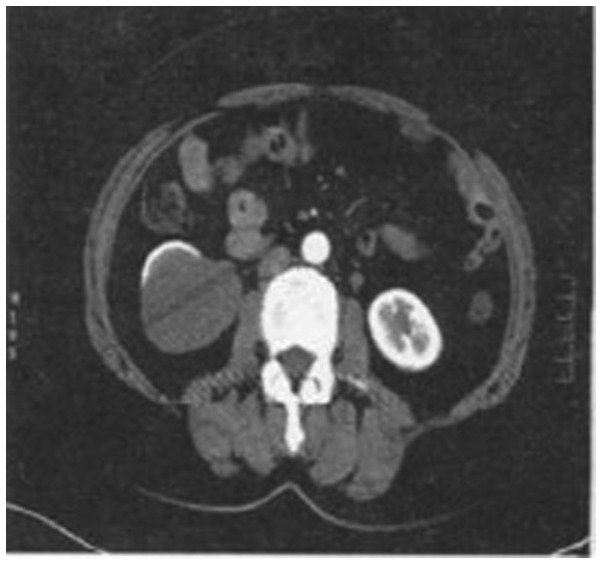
Arterial phase computed tomography. The tumor showed no clear enhancement in this phase.
